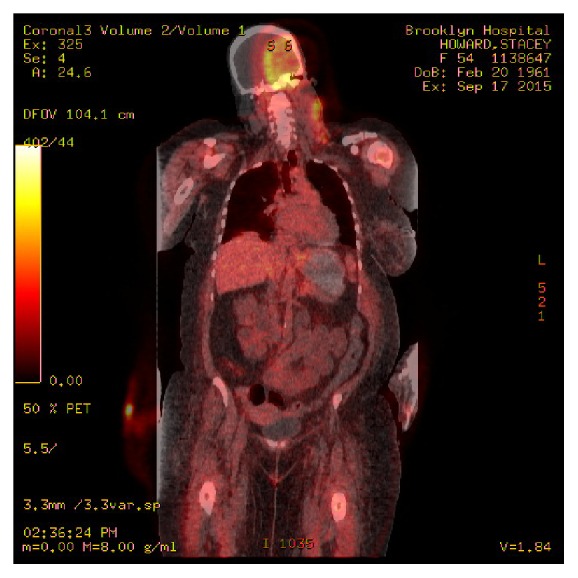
Positron Emission Tomography/Computed Tomography (PET/CT) showing minimal FDG uptake at the large mass lesion at the left ethmoid and maxillary sinuses, moderate to significant FDG uptake at bilateral cervical lymph nodes, and minimal heterogeneous FDG uptake along the thoracolumbar vertebra and pelvic bones.
